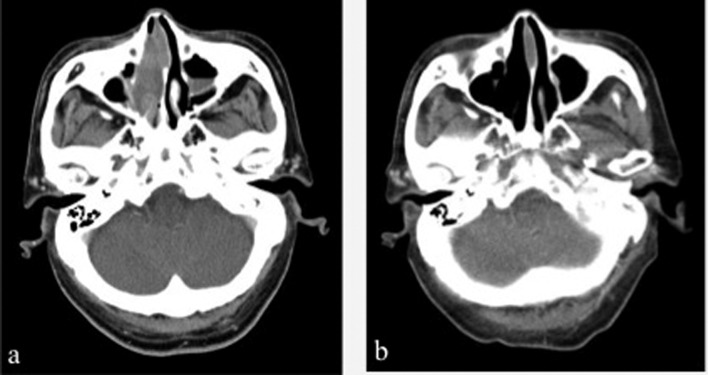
A 72-year-old male received a total dose of 50 Gy and 4 cycles of dexamethasone, etoposide, ifosfamide and carboplatin (DeVIC). (a) Computed tomography (CT) scan prior to chemoradiotherapy (CRT) shows a right nasal space-occupying soft tissue mass. (b) The mass completely disappeared 1 month after the completion of CRT.
